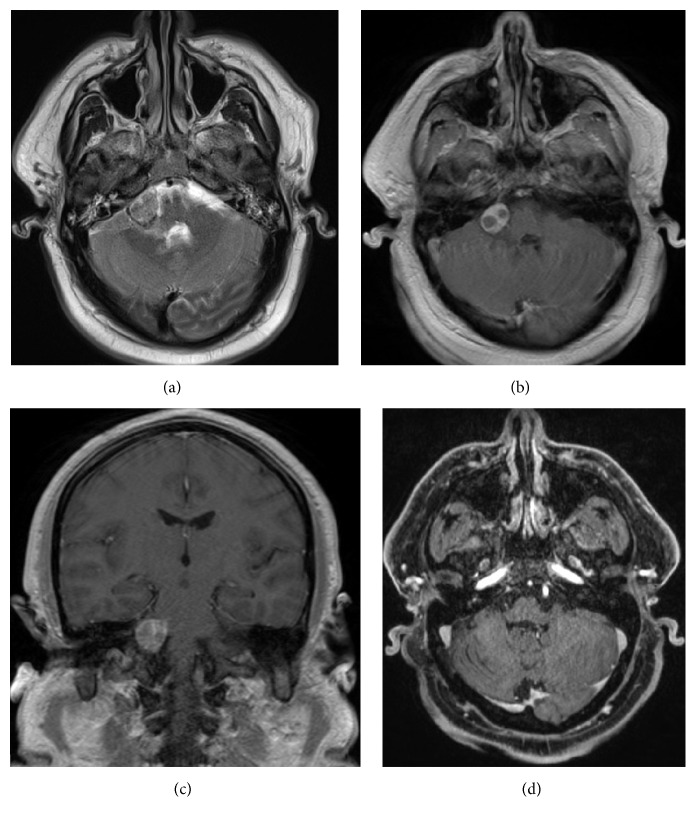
Axial T2-weighted (a) as well as axial (b) and coronal (c) T1-weighted contrast-enhanced magnetic resonance imaging (MRI) of the brain demonstrates an enhanced right cerebellopontine angle mass underlying the hemorrhage in the right cerebellopontine angle with central areas of nonenhancement. One year after resection, axial 3D T1-weighted contrast-enhanced MRI of the brain (d) demonstrates interval postsurgical changes of right retromastoid craniotomy for resection of the right cerebellopontine angle tumor without evidence of residual tumor.
